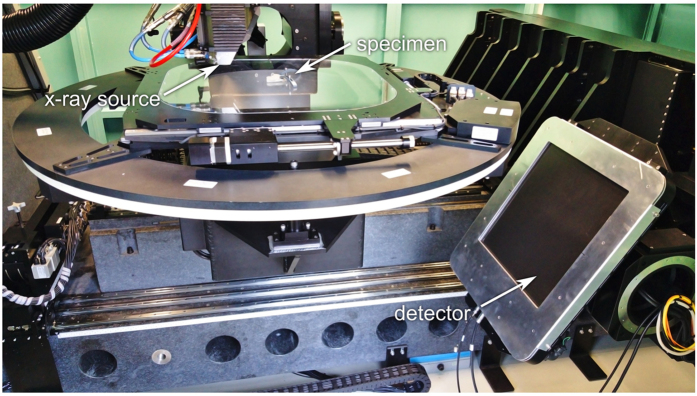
Photograph of the laminography configuration. While the axis of rotation is perpendicular, both x-ray tube and detector are in an inclined orientation, facilitating the laminographic angle of around 29.8°. The distance between source and detector is 1135 mm.
